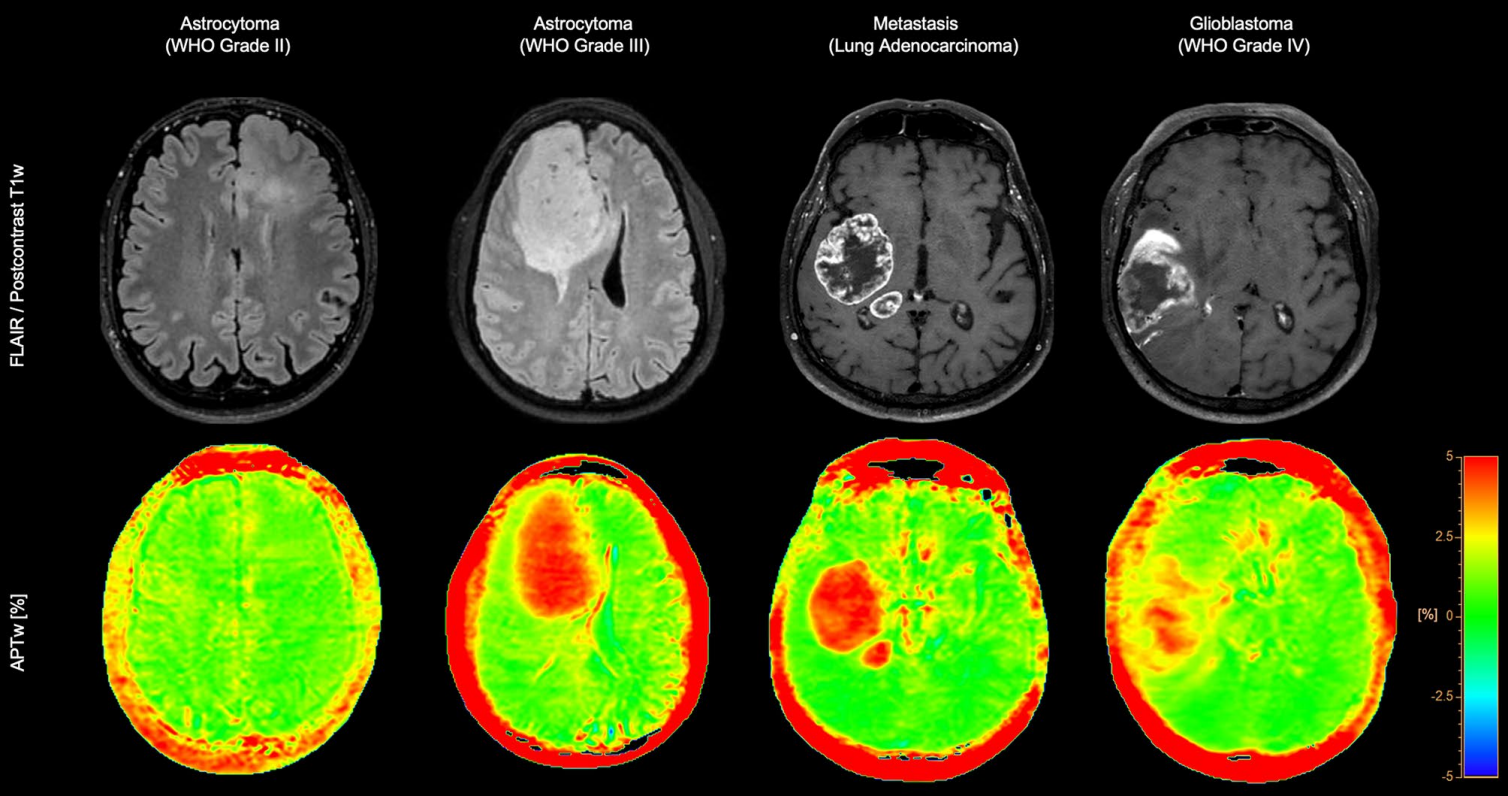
Representative image examples of 4 patients who presented with different neoplasms. FLAIR or T1w postcontrast images are provided in the top row, while the corresponding APTw images are shown in the bottom row.
